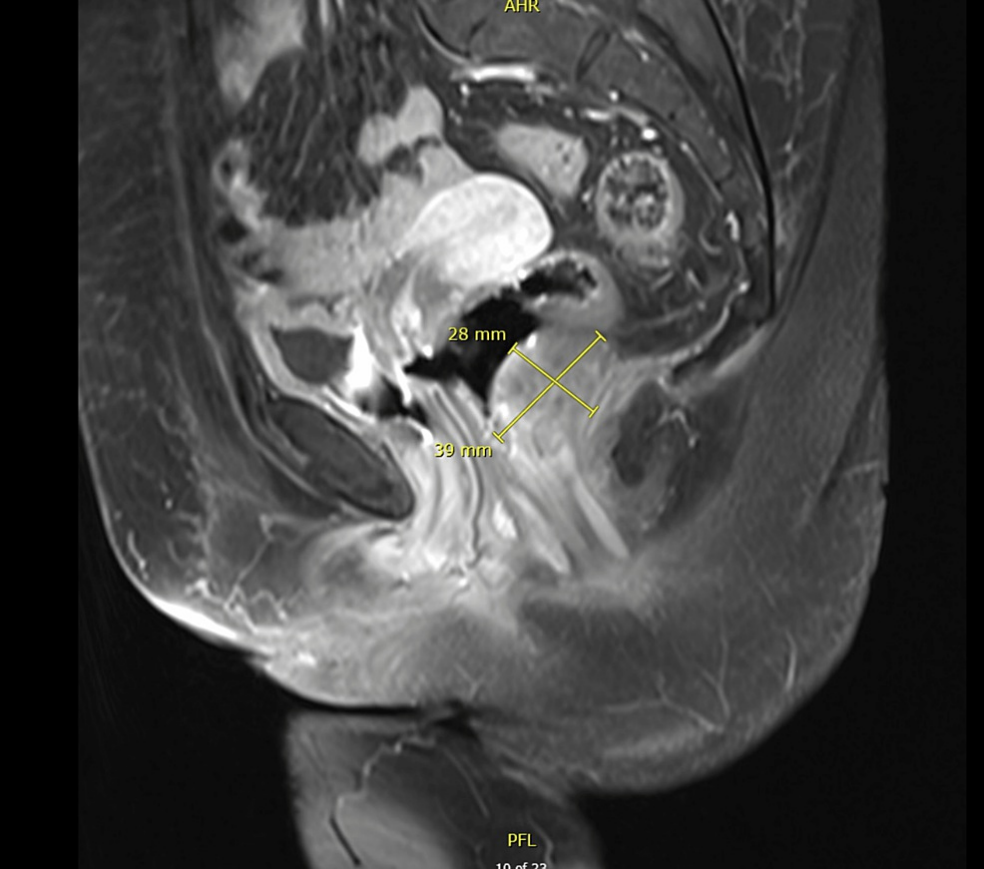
MRI post-neoadjuvant imatinib therapy MRI of the pelvis with saggital view showing the rectal tumor reduction in size to half post-neoadjuvant imatinib therapy
